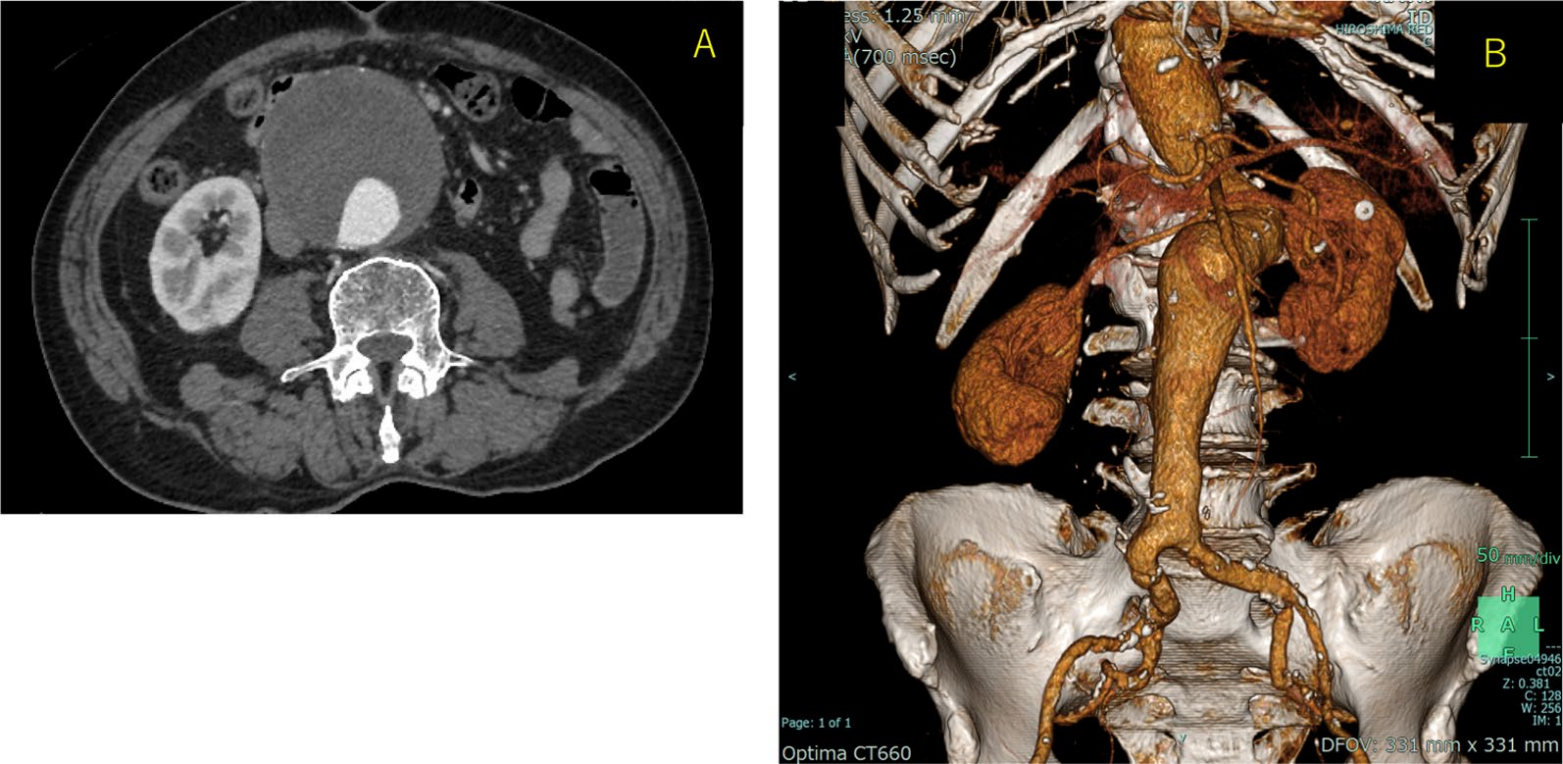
A Preoperative enhanced computed tomography (CT) image showing an 83 mm abdominal aortic aneurysm (AAA) with a large mural thrombus. B Three-dimensional computed tomography image
